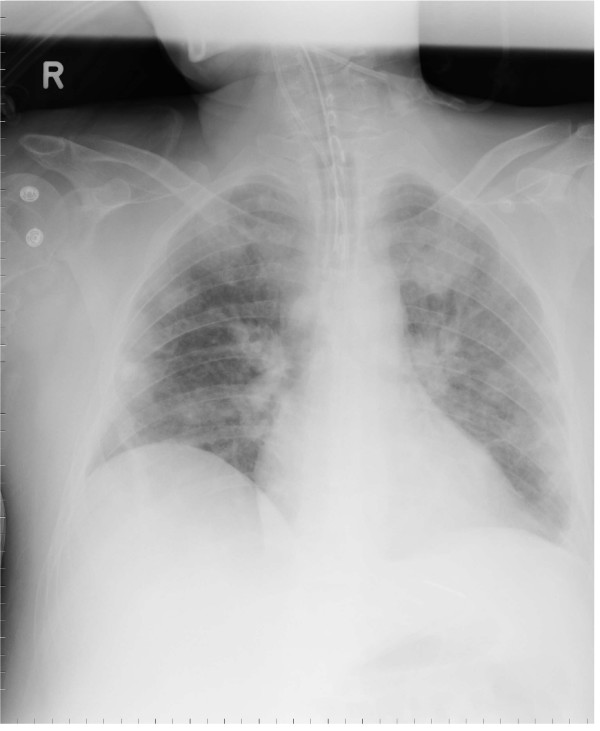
Chest X-ray imaging at admission.
